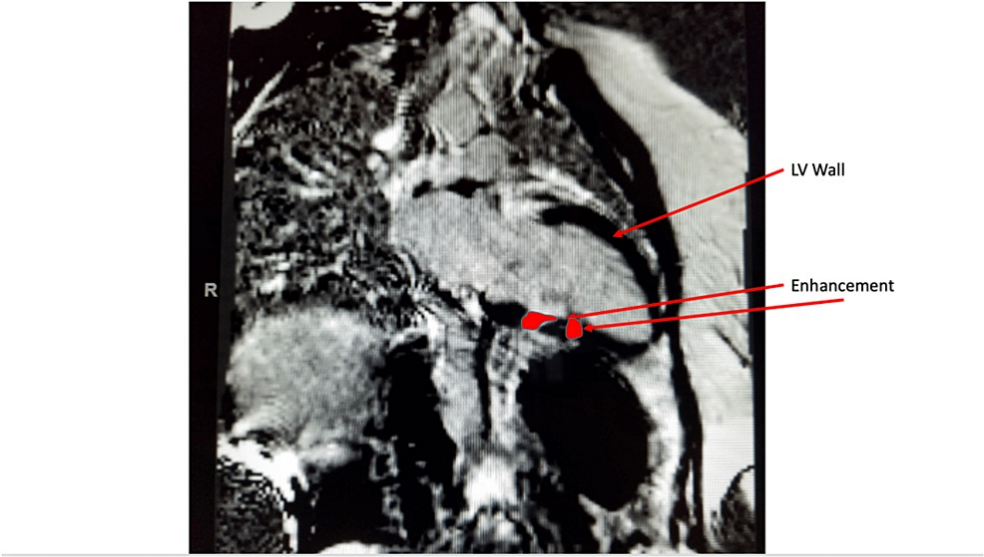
Cardiac MRI. Late gadolinium enhancement in the left ventricular wall.
